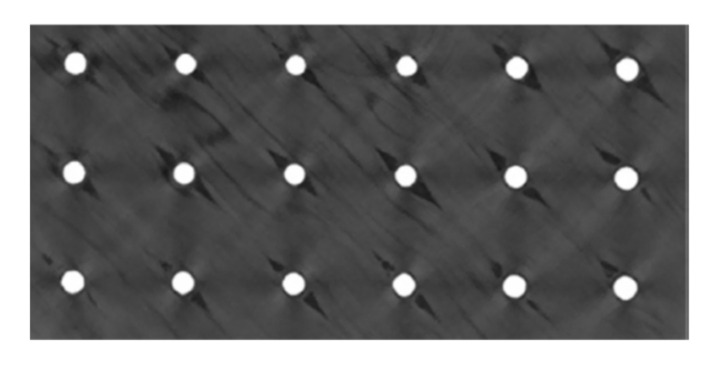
CT picture showing resin rich zones and fibre misalignment around pins [66].
